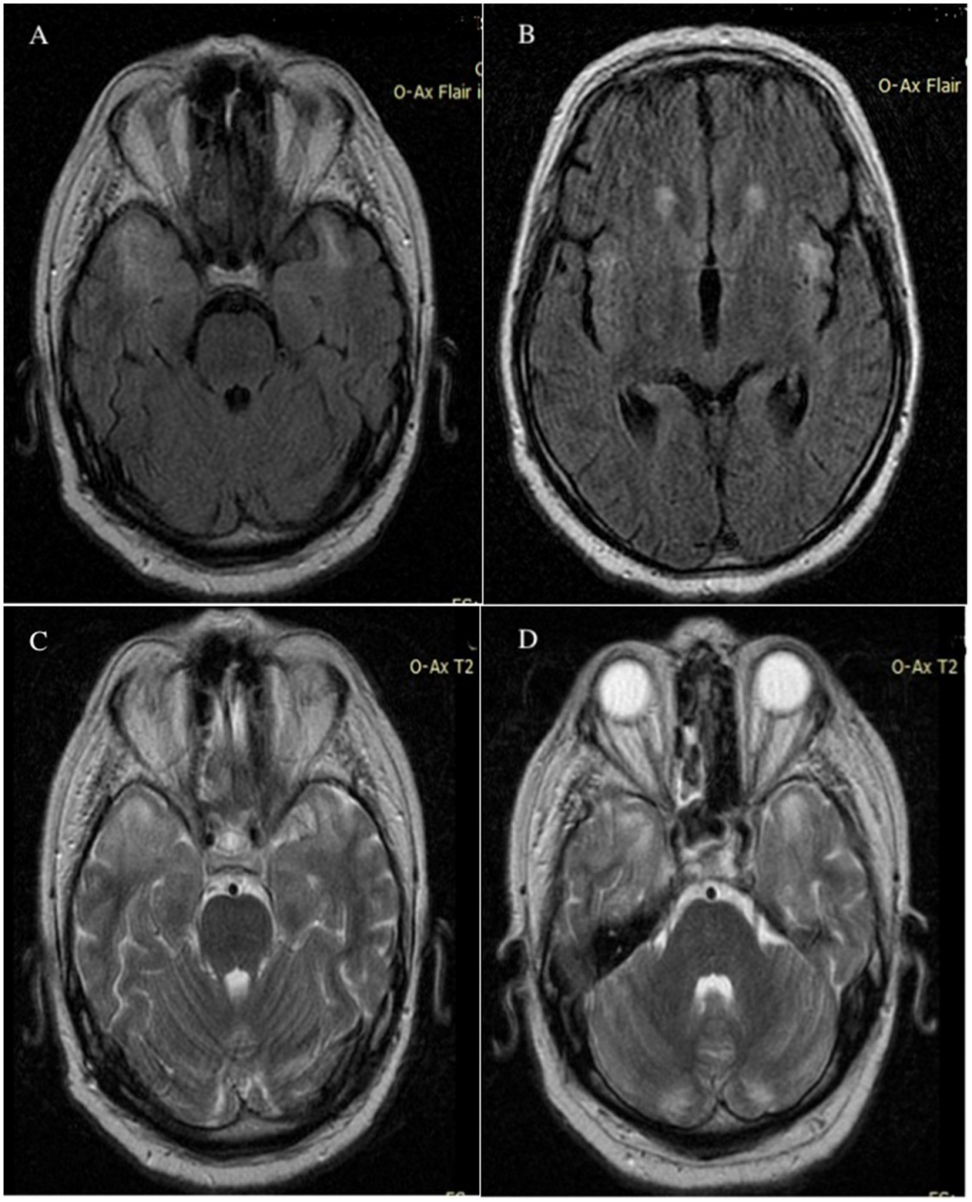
MRI axial Flair FSE images without contrast demonstrated abnormally high signals in the bilateral temporal lobes (A) and in the bilateral insula of the Reil lobes (B) MRI T2 axial images without contrast showed hypersignal in bilateral temporal lobes (C) and cerebellar white matter (D); imaging appearance was unchanged after 6 months. MRI, magnetic resonance imaging; Flair, fluid attenuated inversion recovery; FSE, fast spin echo.
